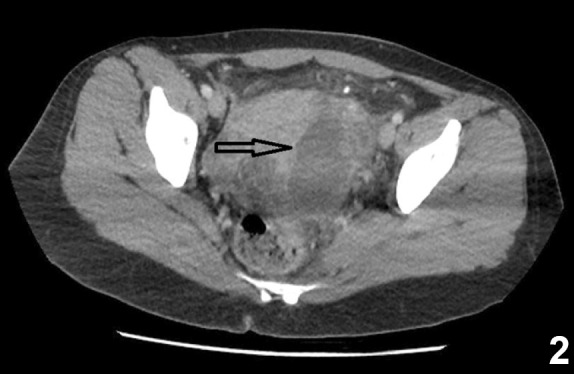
Abdomino-pelvic computed tomography showing a 69×41 mm abscess adjacent to the iliac vascular structures.
